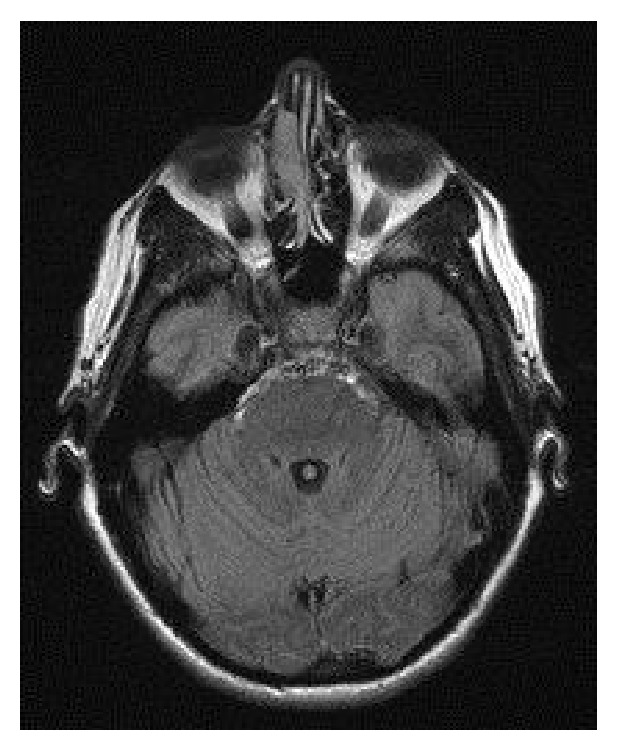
T1-weighted axial MRI demonstrating the mass in the right nasal cavity.
